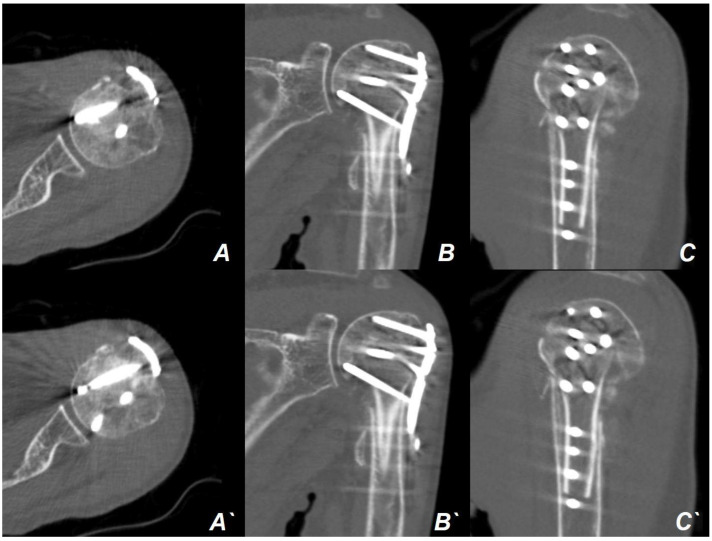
Computed tomography (CT) images performed at the 3-month postoperative follow-up. Progressive bony union was identified in the axial (A,A′), coronal (B,B′), and sagittal (C,C′) slices of the CT images.
